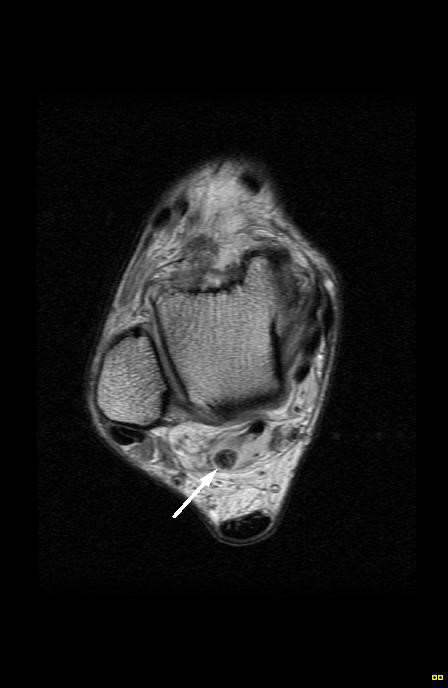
Axial MRI of the right ankle (proton density) revealing a (arrow) heterogeneous nodule of low and intermediate signal intensities located in the flexor hallucis longus tendon sheath. Of interest is the degree of distension of the tendon sheath secondary to the surrounding effusion.
